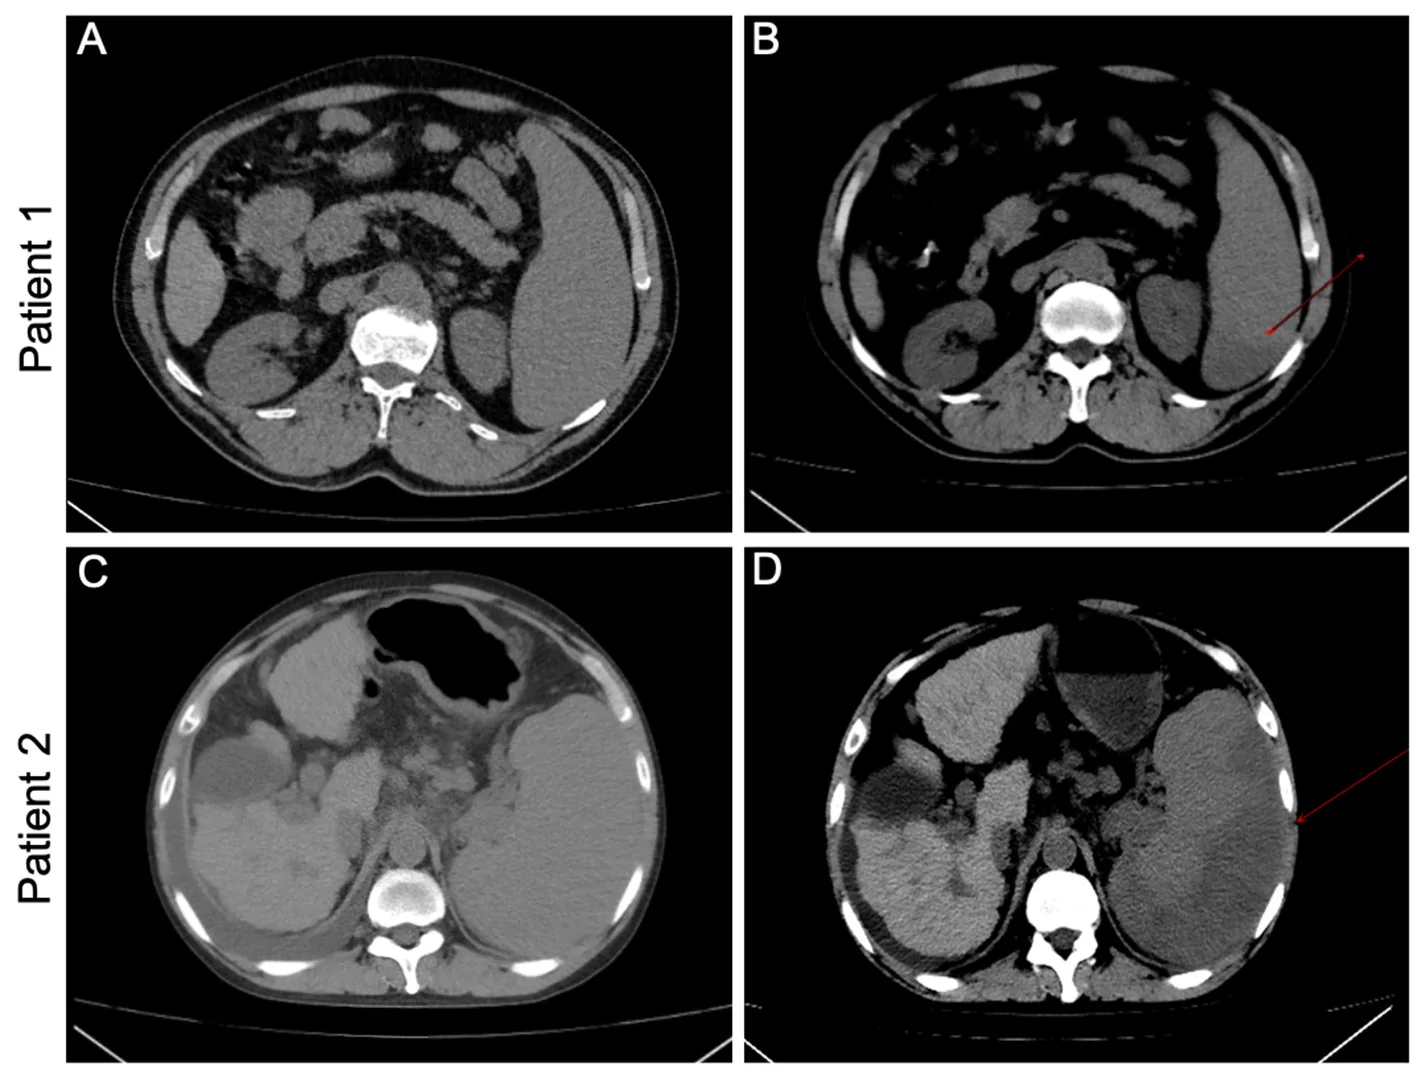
Representative CT images of splenic infarction before and after endoscopic glue injection. (A) Pre-procedural CT of Patient 1 showing mild splenomegaly. (B) Post-procedural CT of Patient 1 demonstrating a wedge-shaped peripheral hypodense infarct (red arrow). (C) Pre-procedural CT of Patient 2 showing marked splenomegaly. (D) Post-procedural non-contrast CT of Patient 2 revealing a large geographic hypodense infarct involving approximately 50% of the splenic volume (red arrow), with minimal peri-splenic fluid.
